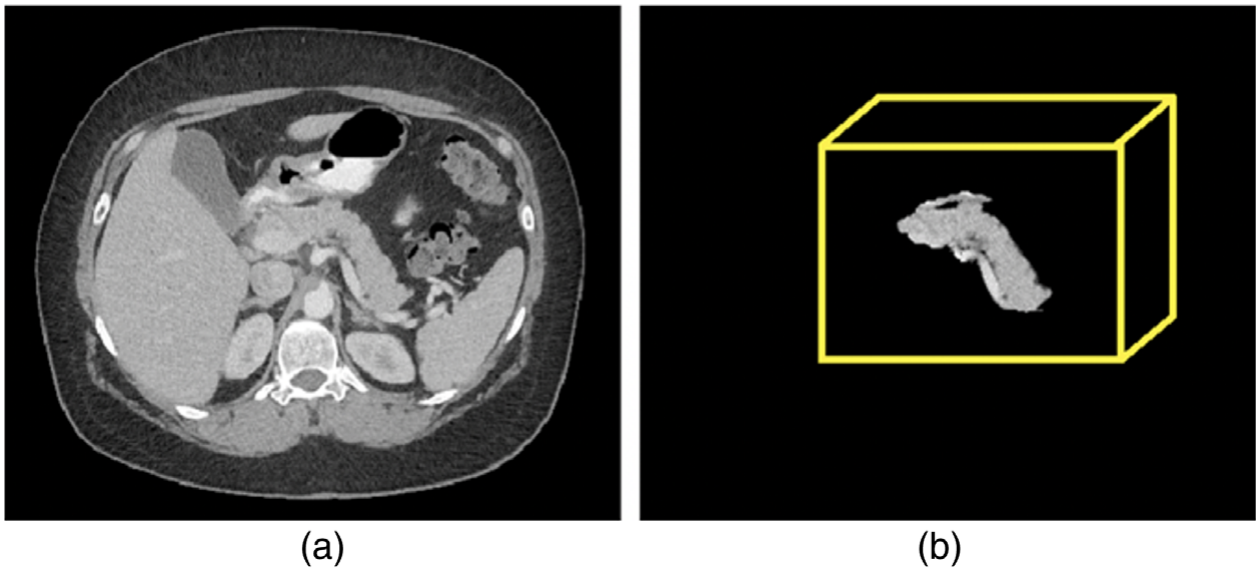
(a) A sample abdominal CT image and (b) the localized 3D pancreas using proposed VGG-19 localizer.
